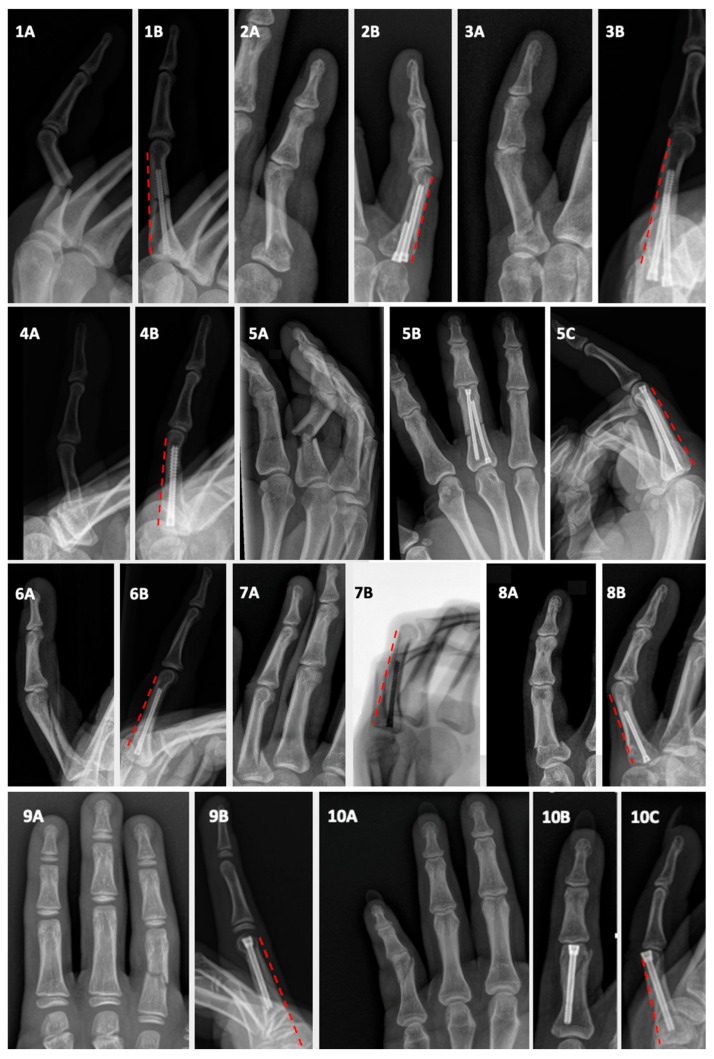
Preoperative and postoperative images of eight patients with anatomically reduced proximal phalangeal fractures with good to excellent TAM. For each patient, panel (A) shows the preoperative radiograph and panels (B,C) show the postoperative true lateral view. In all cases, the dorsal cortex is perfectly aligned without any step-offs (red dot lines), resulting in a smooth gliding surface for the extensor apparatus. (1A,B). A 31-year-old male with a transverse diaphyseal fracture of the right index finger. (1A). Preoperative true lateral view. (1B). Postoperative true lateral view showing antegrade insertion of a single partially threaded IMHCS. (2A,B). 56-year-old female with a base fracture of the left little finger. (2A). Preoperative posteroanterior (PA) view. (2B). Postoperative true lateral view showing two partially threaded IMHCSs inserted antegrade using the double-barrel technique. (3A,B). A 44-year-old female with a base fracture of the left little finger. (3A). Preoperative oblique view. (3B). Postoperative true lateral view showing double-barrel antegrade screw fixation. (4A,B). A 60-year-old female with a proximal-third fracture of the left little finger. (4A). Preoperative true lateral view. (4B). Postoperative true lateral view showing a single fully threaded IMHCS inserted antegrade. (5A–C). A 48-year-old male with a diaphyseal fracture of the right middle finger. (5A). Preoperative oblique view. (5B). Postoperative PA view. (5C). Postoperative true lateral view showing two partially threaded IMHCSs inserted using the technique of the double-opposing screws. (6A,B). A 15-year-old male with a spiral fracture of the left ring finger. (6A). Preoperative PA view. (6B). Postoperative true lateral view showing antegrade insertion of a single fully threaded IMHCS. (7A,B). A 20-year-old male with a base fracture of the left ring finger. (7A). Preoperative and (7B). postoperative true lateral views showing antegrade insertion of a single fully threaded IMHCS. (8A,B). A 41-year-old female with a base fracture of the left little finger. (8A). Preoperative PA view. (8B). Postoperative true lateral view showing antegrade insertion of a single partially threaded IMHCS. (9A,B). A 12-year-old male with a straight diaphyseal fracture of the right ring finger. (9A). Preoperative view. (9B). Postoperative true lateral view showing retrograde insertion of a single fully threaded IMHCS. (10A–C). A 15-year-old male with a distal metaphyseal fracture of the right little finger. (10A). Preoperative PA view. (10B). Postoperative PA view. (10C). Postoperative true lateral view showing retrograde insertion of a single partially threaded IMHCS.
